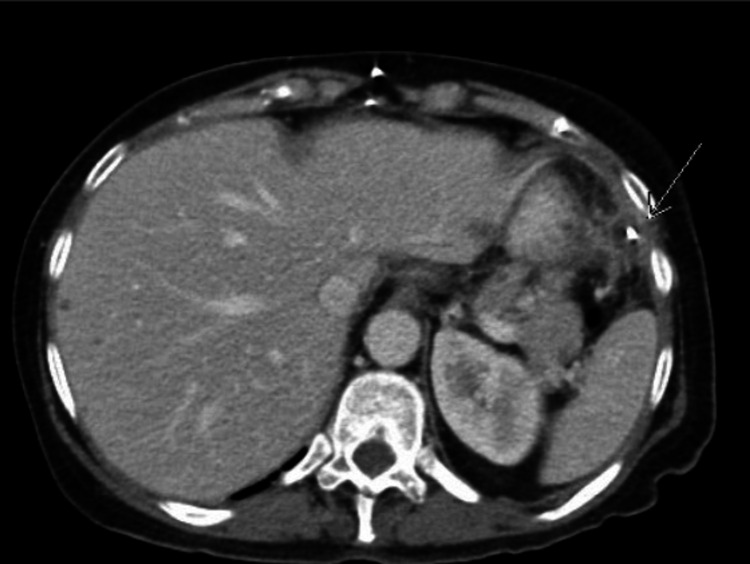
CT abdomen and pelvis with contrast showing fluid collection hypodense around the VP shunt denoted with a white arrow.
